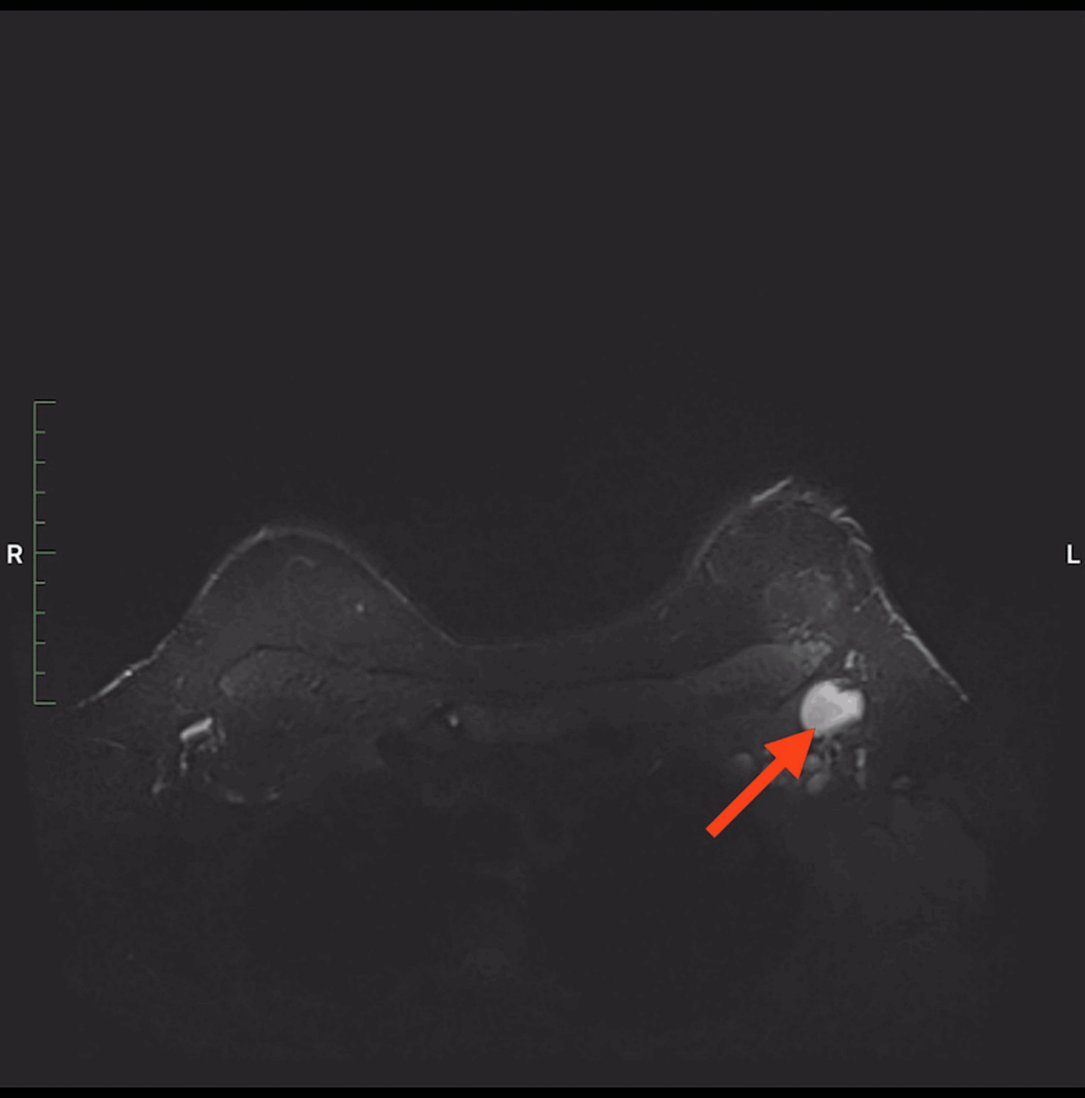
MRI of both breasts showing the left axillary node (red arrow) MRI: magnetic resonance imaging
